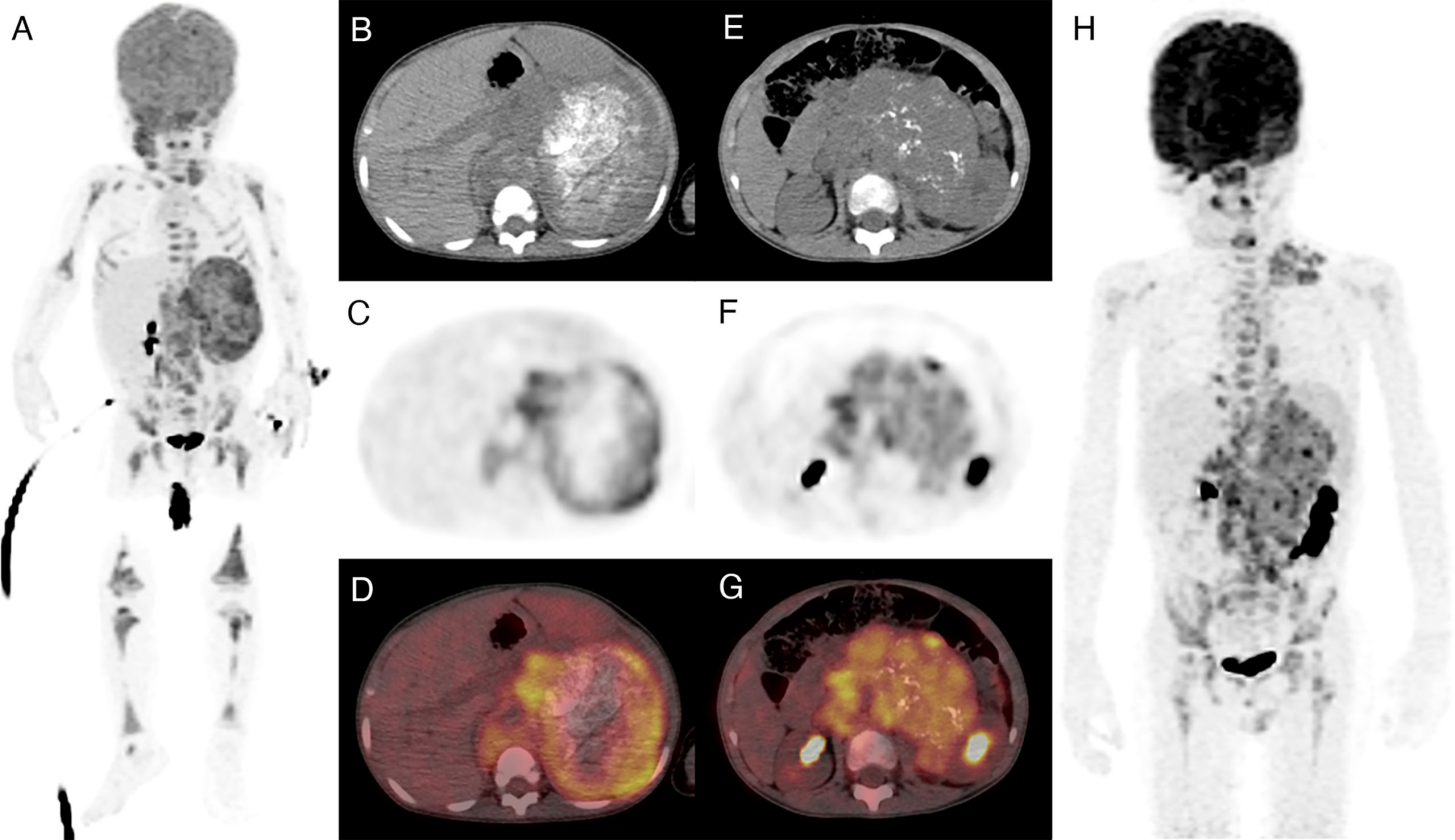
Two patients with a high-risk neuroblastoma and high or low intratumor heterogeneity. Both patients had amplified MYCN and stage 4 diseases. (A–D) A 20-month-old girl with a highly heterogeneous FDG uptake in the primary tumor (high rad-risk). She progressed 21 months after diagnosis. (E–H) A 5-year-old boy with a relatively homogeneous FDG uptake (low rad-risk). The patient remained recurrence free within 5 years of follow-up.
